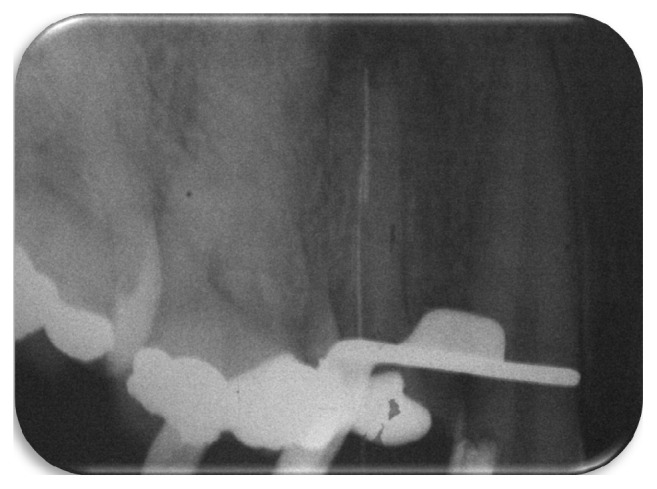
Periapical radiograph of the moment when trying to bypass the fractured instrument using manual files.
